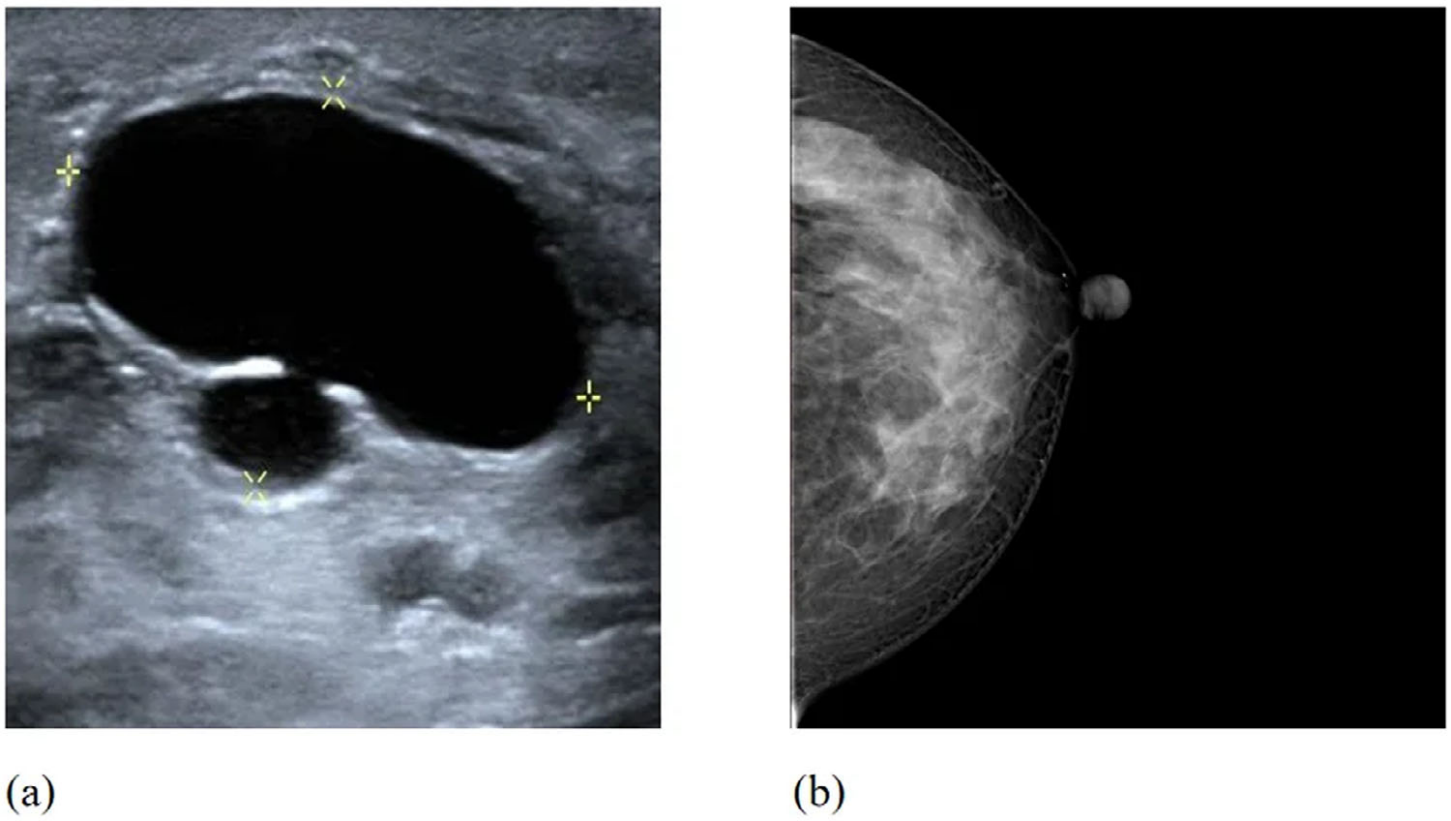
Cropped breast tissue regions used as model inputs: (a) ultrasound breast region containing tumor, (b) mammography breast region containing tumor.
